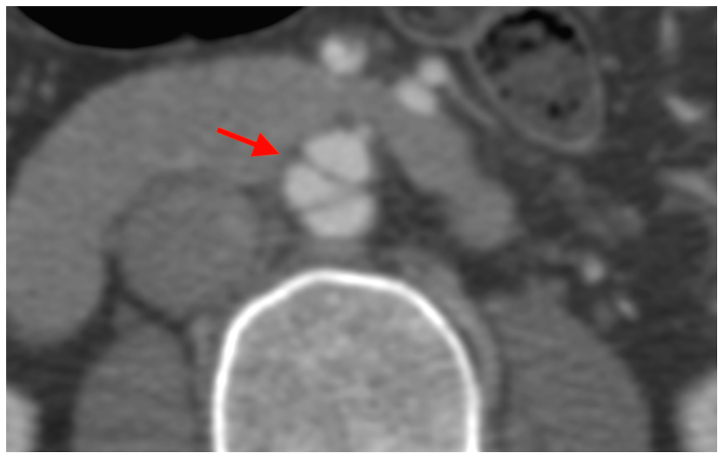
Computed tomography (CT) angiography of the current clinical presentation. The *red arrow* indicates progression of the dissection with aneurysmal dilatation and transformation into a triple-lumen configuration, also known as the Mercedes-Benz sign.
